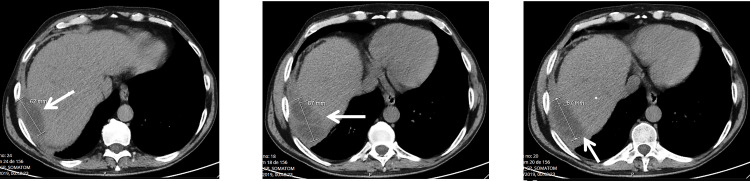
Abdominal CT scan performed upon admission to the emergency department With an arrow, the newly identified hepatic lesion is indicated, and its dimensions are marked in millimeters.
